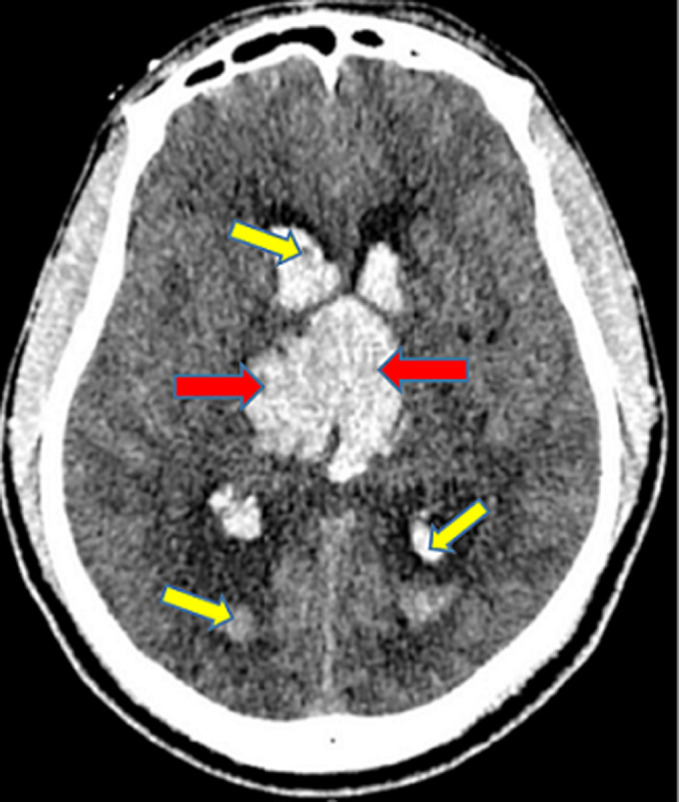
an axial non-enhanced CT scan of the brain showing hyperdense collections of acute bleed in both thalami (red arrows) and extension into the lateral ventricles (yellow arrows)
